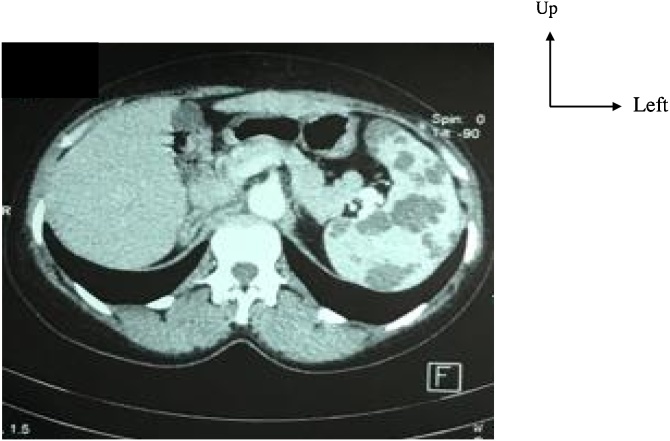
Abdominal CT scan showed multiples cystic lesions hypodense without enhancement. The spleen size is normal.
